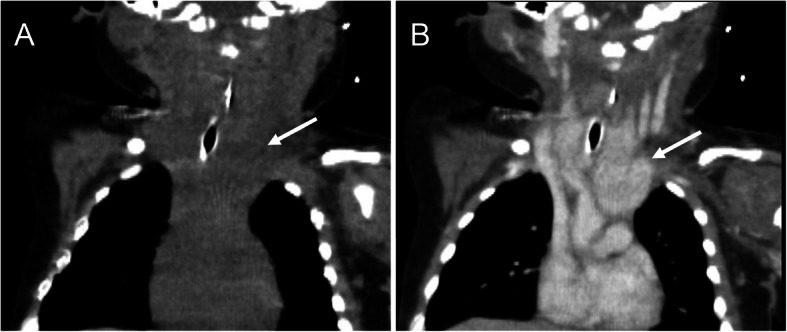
Non-enhanced and contrast-enhanced CT images of the thyroid hemangioma. a Non-enhanced CT imges show that the slightly hyperattenuated thyroid is enlarged and part of the left lobe stretches into the superior mediastinum. b In the contrast-enhanced CT image, the enlarged thyroid shows heterogeneous nodular enhancement
